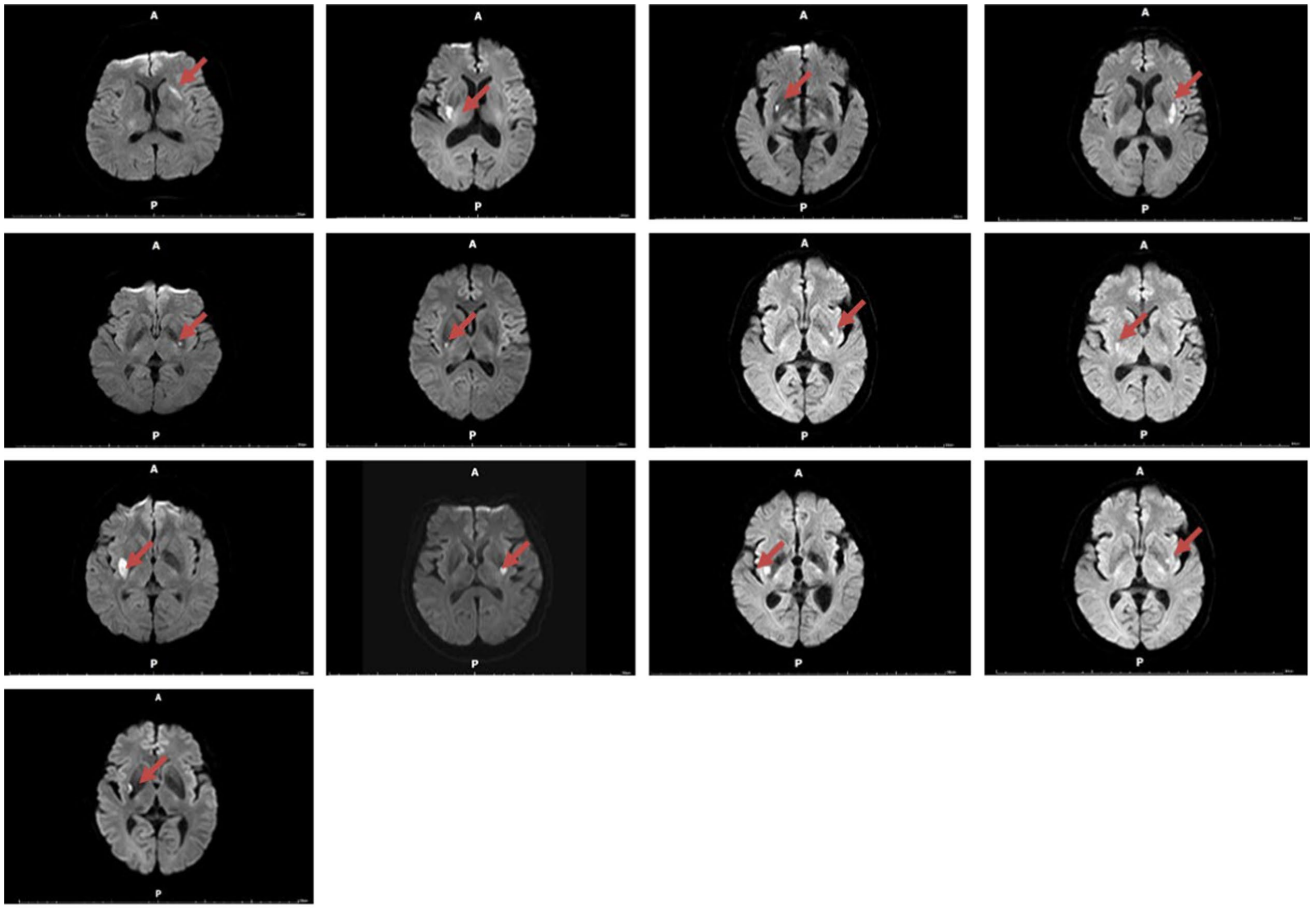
Magnetic resonance imaging (diffusion-weighted imaging, DWI) was performed on 13 smokers with dorsal striatum damage.
